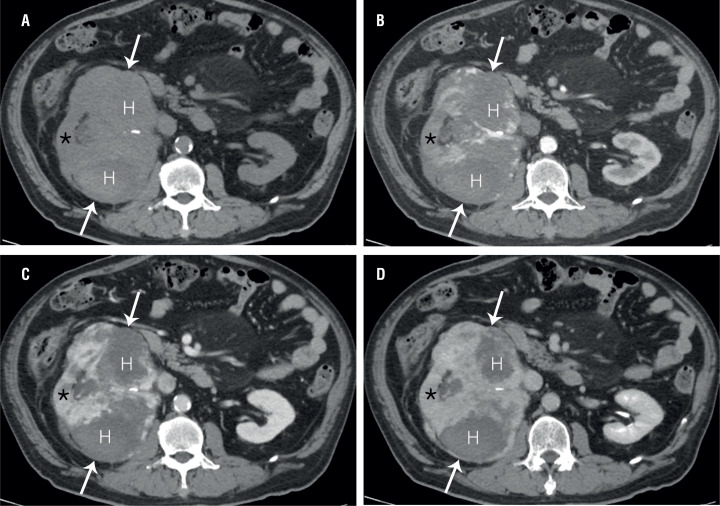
Axial CT images of pre-contrast (A), contrast-enhanced corticomedullary (B), nephrographic (C) and excretory (D) phases depict a huge mass (arrows) with extensive hemorrhage (H) in the right kidney, which shows progressive peripheral nodular enhancement with a delayed fill-in. Note the presence of residual renal parenchyma (*).
